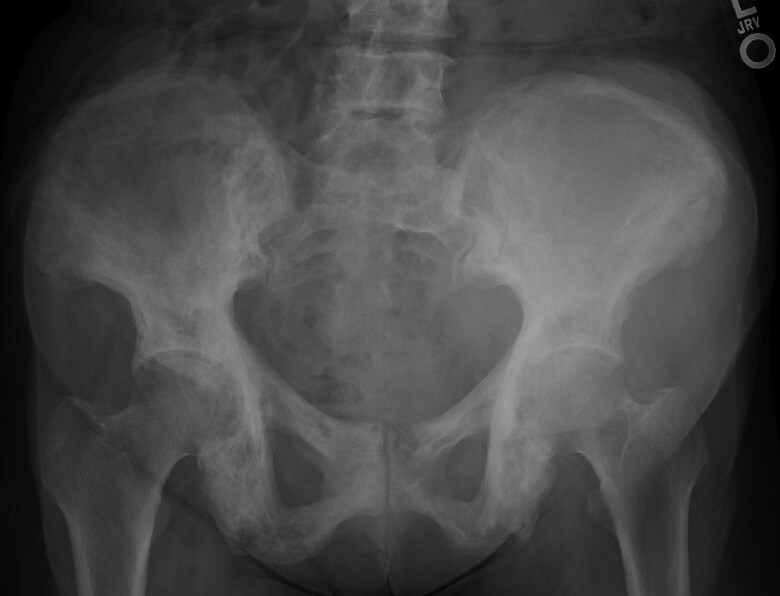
Pelvic x-ray with coarse trabecula and cortical thickening in bilateral pelvic bones suggest Paget disease.
